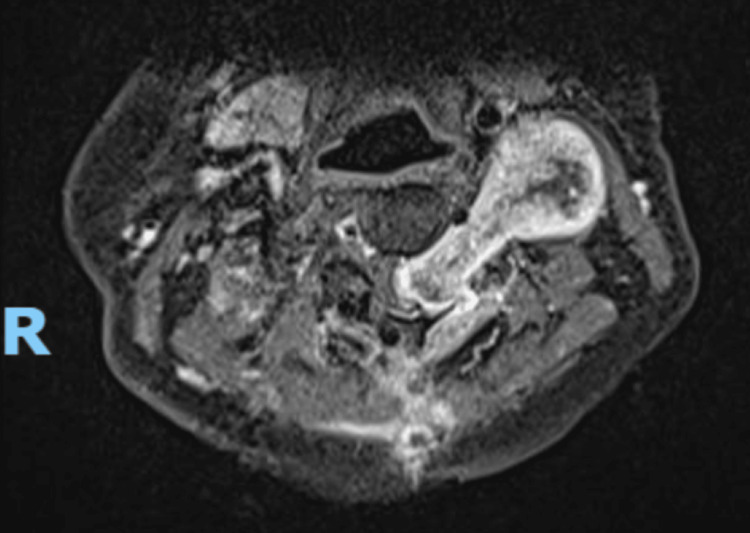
Pre-operative MRI prior to second surgery suggesting tumour recurrence.
